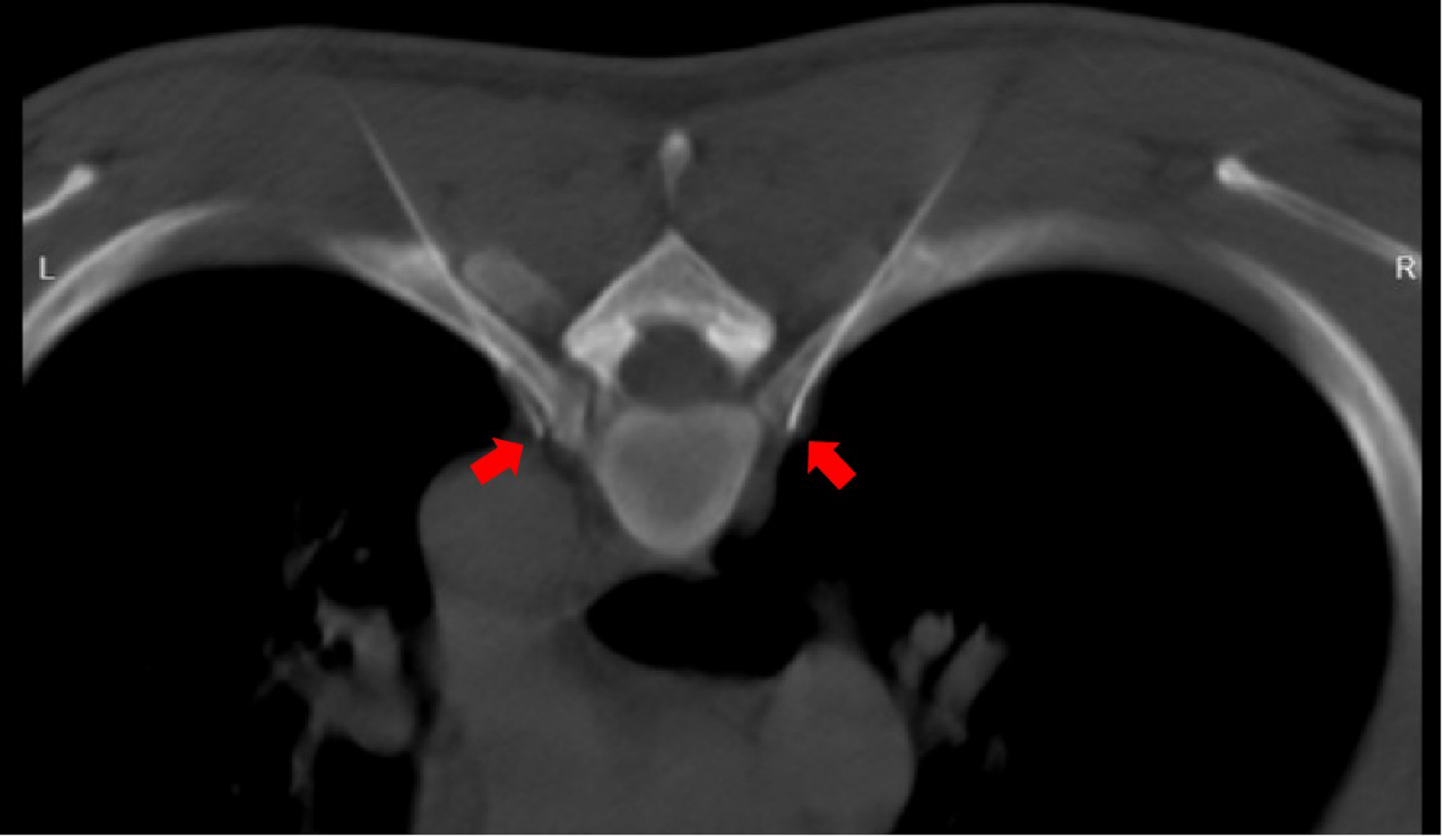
CT-guided puncture reaching the predetermined target point along the designed path (indicated by the red arrow).
